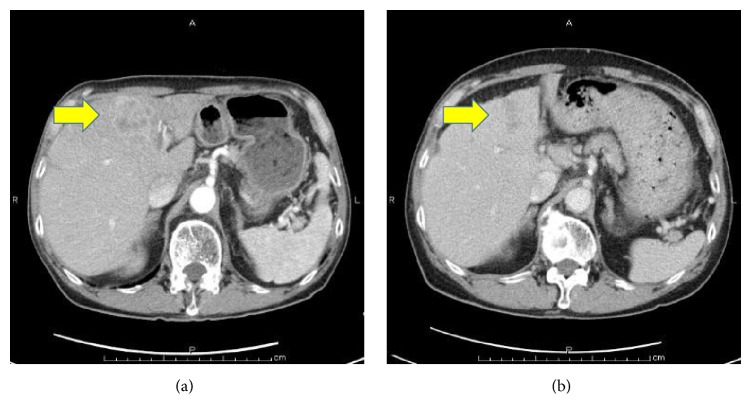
CT scans showed that the patient's liver tumors (arrows) were reduced by the 78th (a) and 238th day (b) of admission after chemotherapy with CDDP and GEM.
